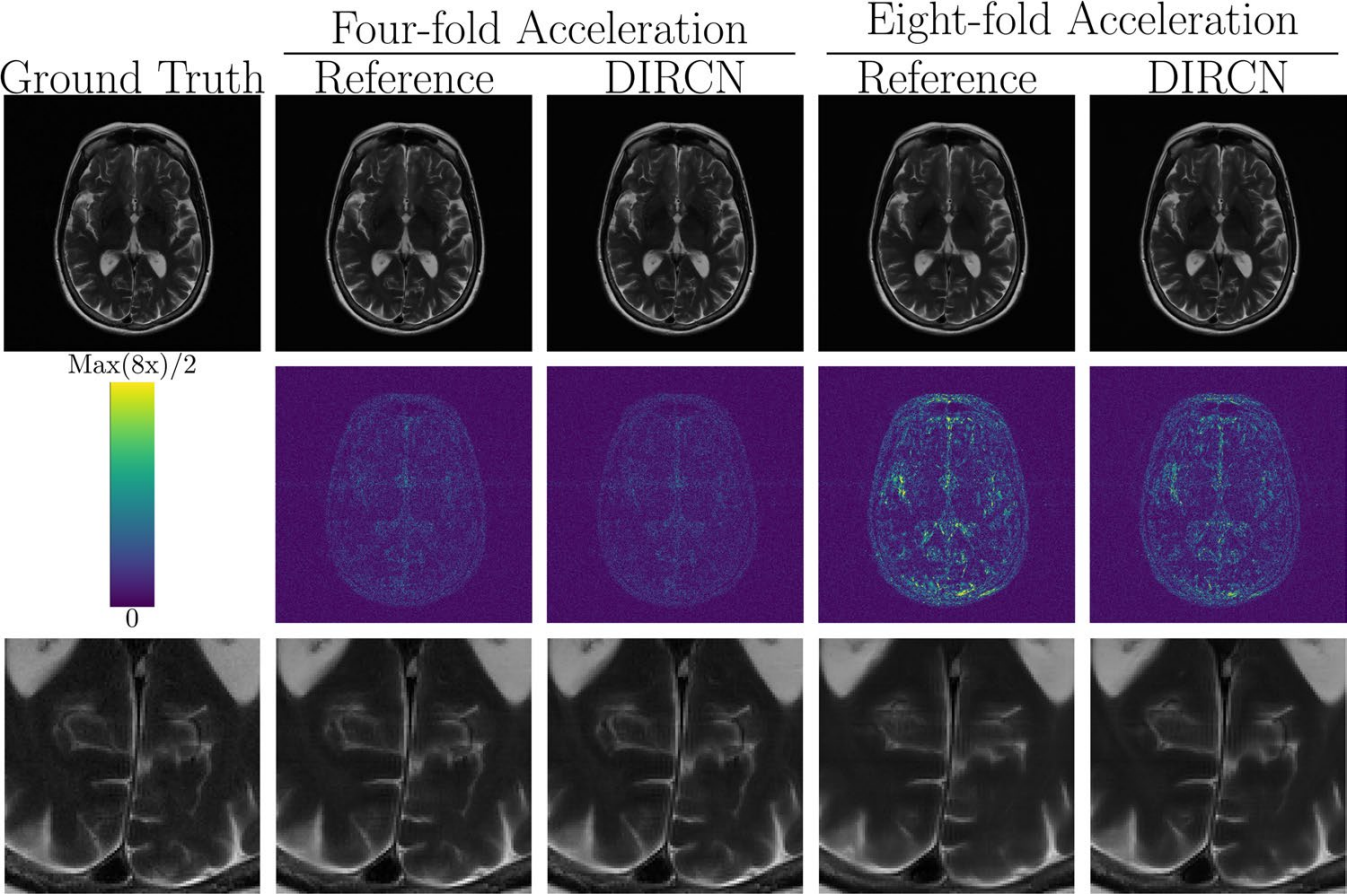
A representative example of a T2-weighted reconstruction with the reference model and DIRCN for four- and eightfold acceleration. This includes their respective reconstructions and the corresponding error map (absolute difference) between the fully sampled image and the reconstruction. The colormap goes between 0 and half the maximum error for eightfold acceleration to emphasize visual difference. The bottom images are a region of interest where differences between the reference model and DIRCN reconstructions for eightfold acceleration can be seen
